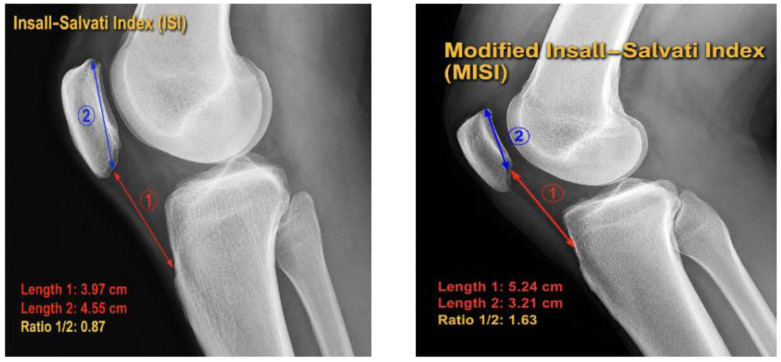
X-ray assessment of patellar height.
